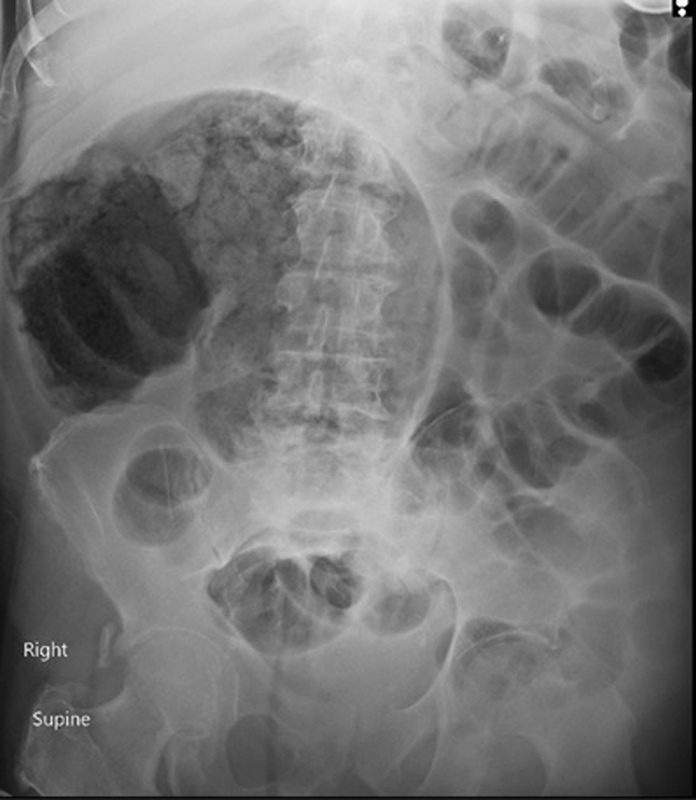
Abdominal X-ray showing dilated large bowel loop in the right side of the abdomen.
